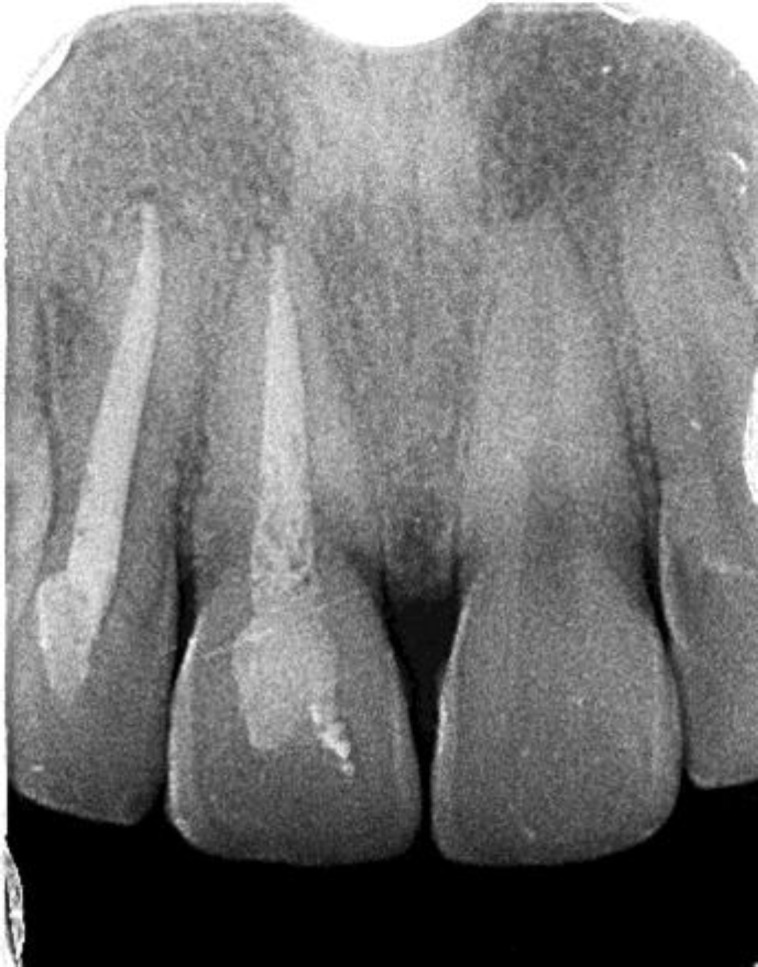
Radiograph at 3-year follow-up
